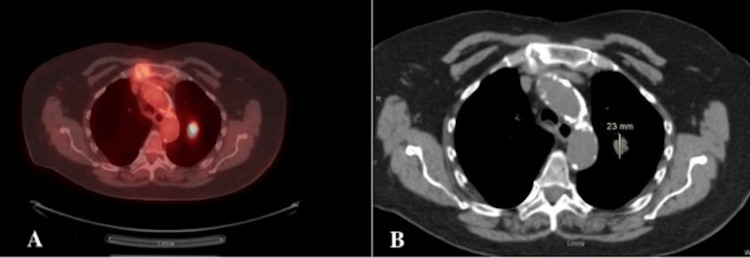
Axial positron emission tomography-computed tomography (PET-CT) scan images. (A) Axial PET-CT scan shows a PET-avid lesion in the left upper lobe of the lung, indicating increased metabolic activity, with a standardized uptake value (SUV) of 7.3, staged as cT1cN0M0 stage IA. (B) Axial non-contrast CT scan of the chest shows a 2.3 cm lesion in the left upper lobe, corresponding to the area of interest seen on the PET-CT.
